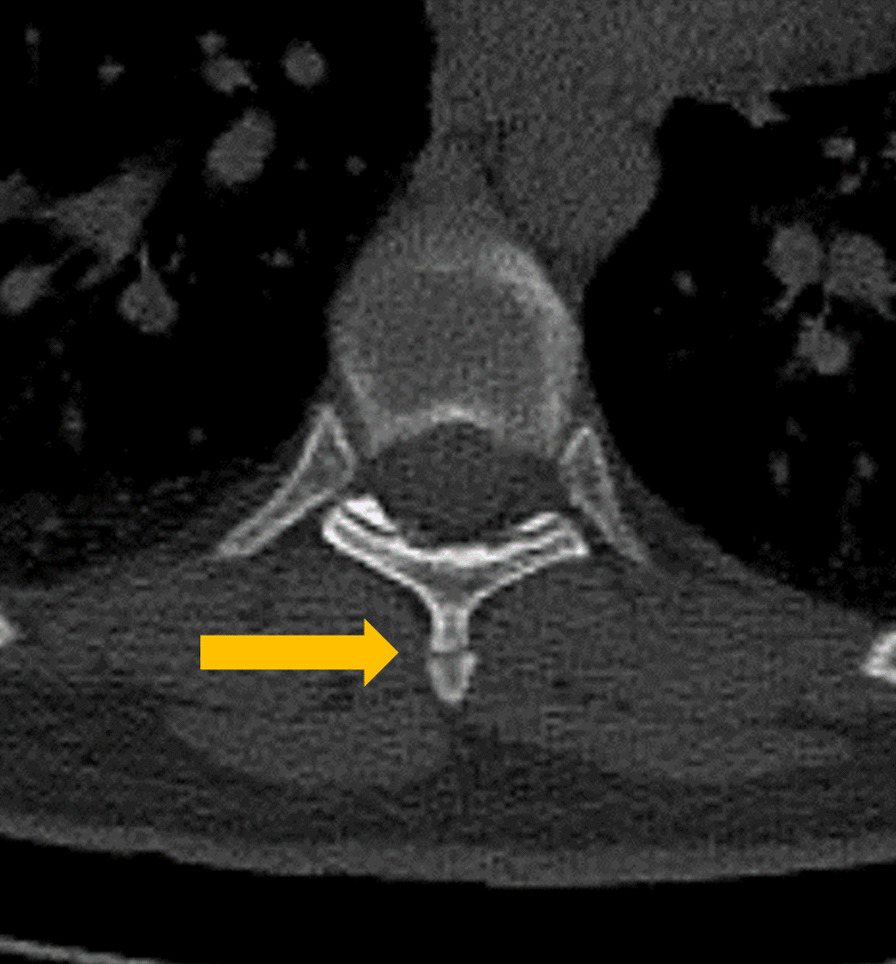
Computed tomography axial view showing a T6 spinous process nonunion (gold arrow) 2 years after the fracture
